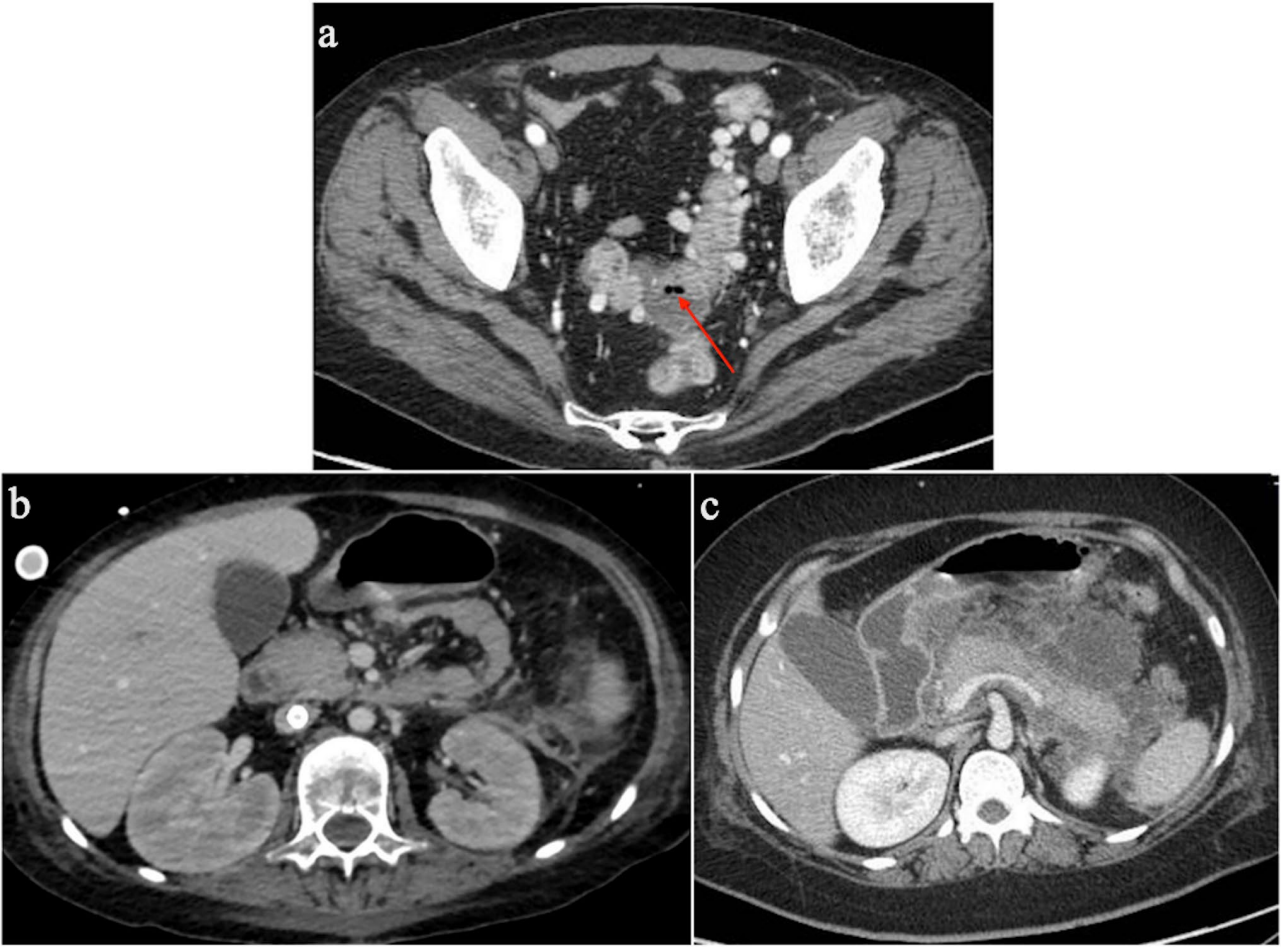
Potential abdominal complications of COVID-19 in patients treated with VV-ECMO. a Axial CT image shows diverticulitis complicated by a peridiverticular abscess with covered bowel perforation (arrow). b Axial CT image obtained in a patient with multiple organ failure and suspected abdominal bleeding. Notice initial swelling of both kidneys with loss of cortico-medullary differentiation. c Axial CT image obtained in a patient with peripancreatic fluid collections from acute pancreatitis
